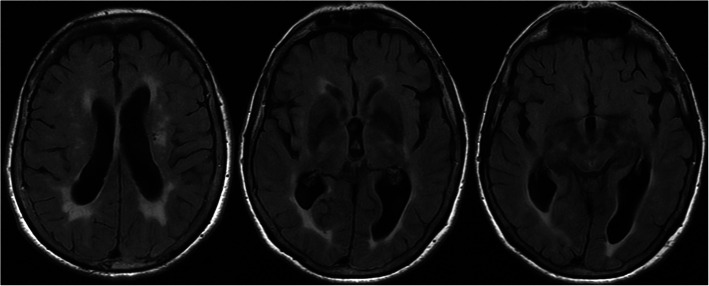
Axial T2-FLAIR brain MRI: left anterior choroid stroke sequelae and significant peri ventricular vascular white matter hyperintensities without other abnormal findings
